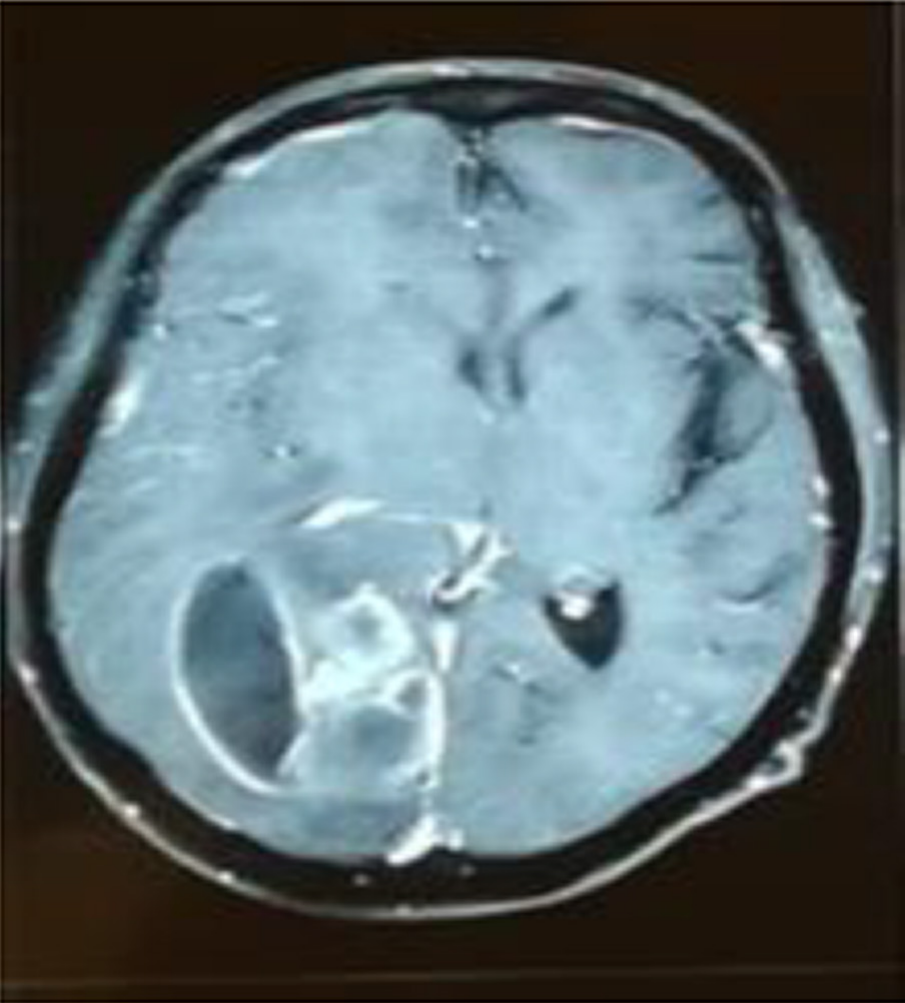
CT SCAN: Effacement furrows and brain fissures in the right temporal–parietal area. Homogeneous hypodense intra‐parenquimatous area, no vascular compromise is visible, with deviation of the midline structures and compressive effect
